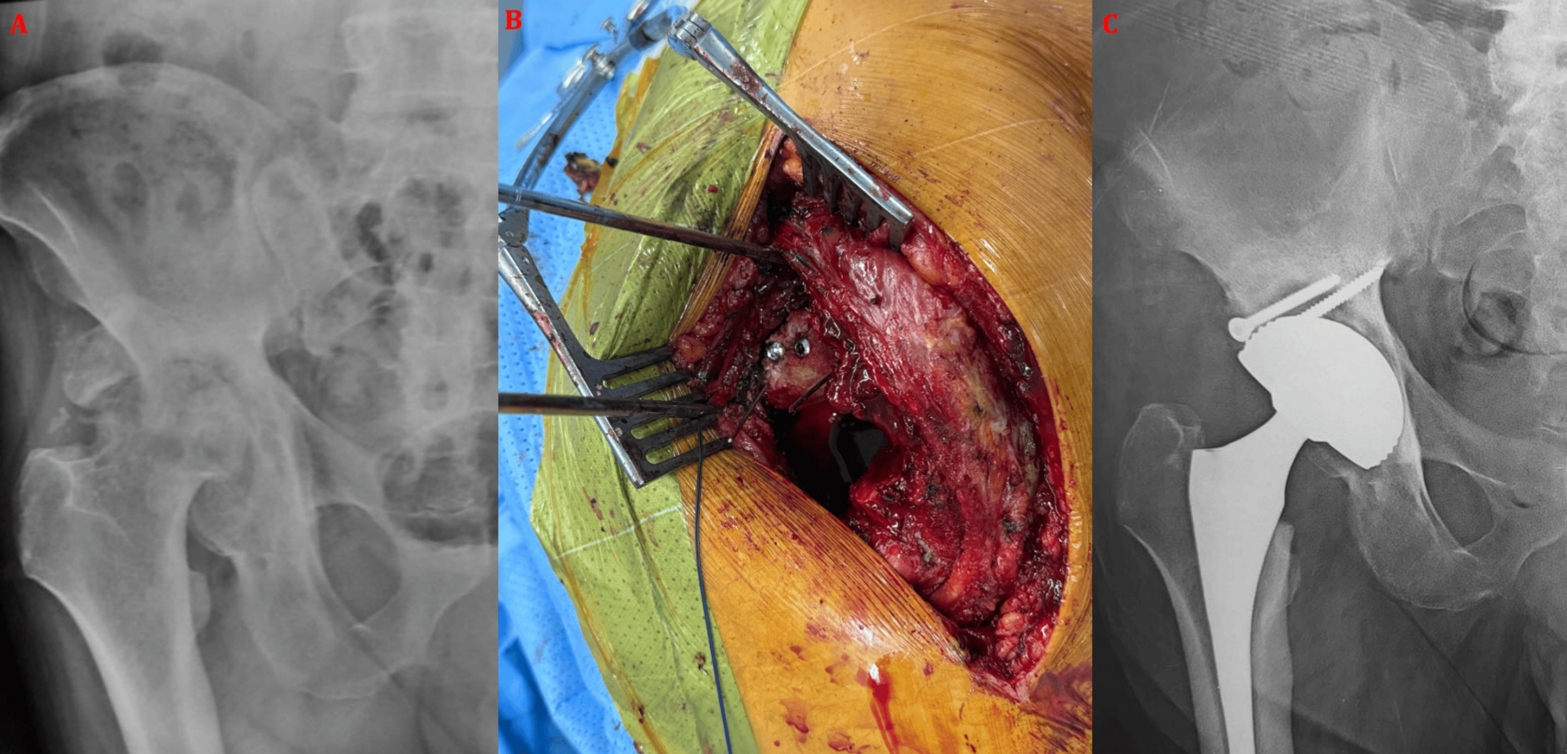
Anteroposterior radiograph of the right hip showing avascular necrosis of the femoral head associated with bone defects of the acetabular roof and posterior wall, as sequelae of a right acetabular fracture initially treated conservatively in a 49-year-old male patient (A). A structural bone allograft was used to fill the acetabular defect and fixed with two screws (B). The postoperative control radiograph demonstrates implantation of the total hip arthroplasty after graft fixation (C).
